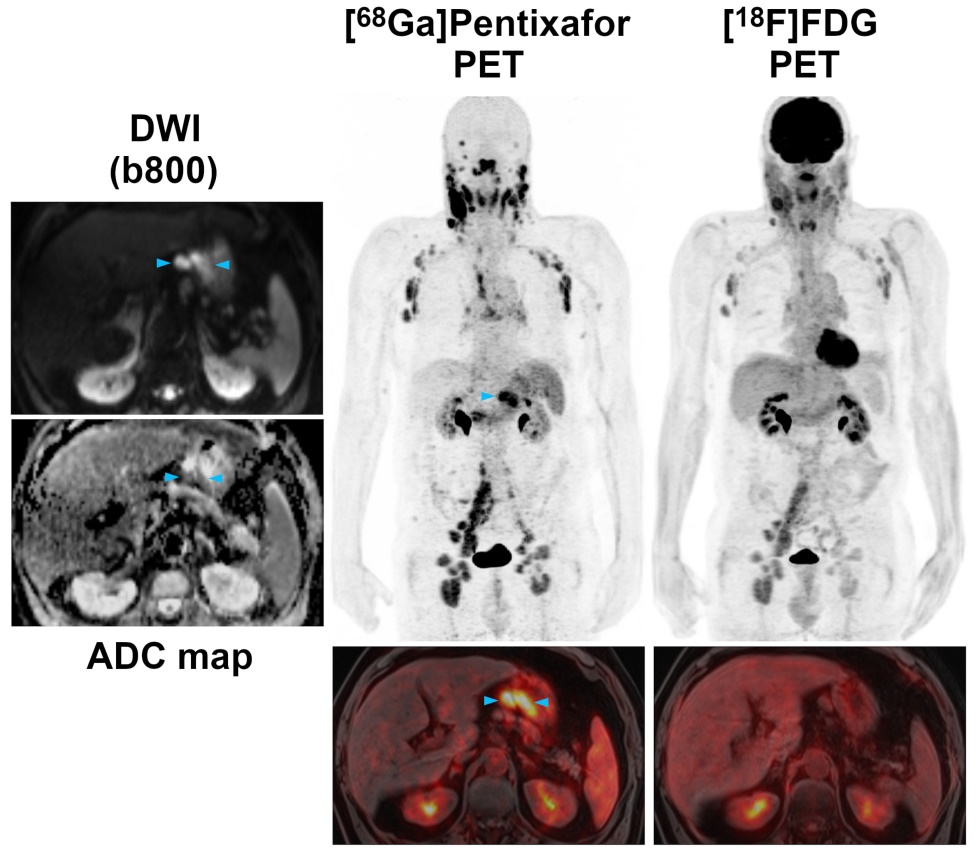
Pre-therapeutic [^68^Ga]Pentixafor-PET/MRI and [^18^F]FDG-PET/MRI of a 61-year-old male MCL patient. While the majority of supra- and infradiaphragmatic nodal lymphoma manifestations show both an increased [^68^Ga]Pentixafor and [^18^F]FDG uptake, the extranodal gastric lymphoma manifestation and an adjacent enlarged lymph node (blue arrowheads) are only positive on [^68^Ga]Pentixafor-PET, but not on [^18^F]FDG-PET. Diffusion-weighted MRI (DWI) confirmed the presence of both lesions, which show a low signal on the apparent diffusion coefficient (ADC) map, reflecting the high cell density typically observed in lymphomas.
